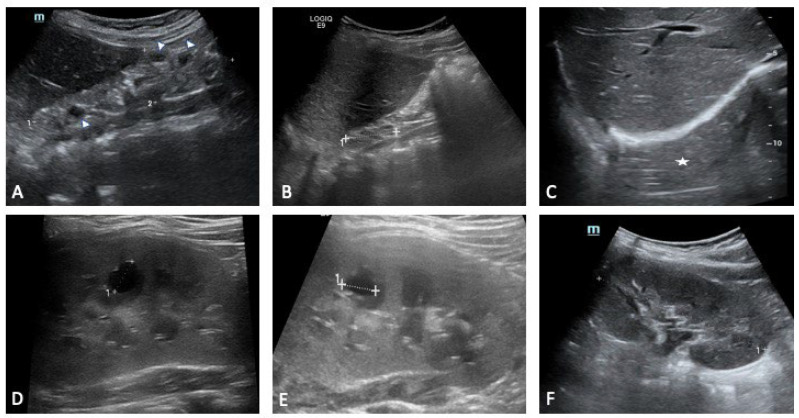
Sibling 1. Abdominal ultrasound examinations were performed at 3 (A,D), 4 (B,E), and 7 (C,F) years of age. (A–C) show progressive right kidney atrophy [maximal pole-to-pole diameter approximately 49 mm (dotted line 1 in (A)) and 33 mm (dotted line 1 in (B)); in (C), the right kidney is not clearly identifiable at the ultrasound exam («empty perirenal space», white asterisk)]. Renal parenchyma structure is altered, diffusely hyperechoic, with no clear differentiation between cortex and medulla (A,B). Some small cystic lesions can also be noted (arrowheads in (A)). Dotted line 2 in (A): antero-posterior diameter (20 mm). (D–F) show progressive compensatory hypertrophy of the left kidney [maximal pole-to-pole diameter approximately of 78 mm, 85 mm and 93 mm, respectively (the latter shown as dotted line 1 in (F))]. Some cystic lesions are identified in the renal parenchyma, with the most prominent of around 10 mm located in the middle third (dotted lines 1 in (D,E)).
